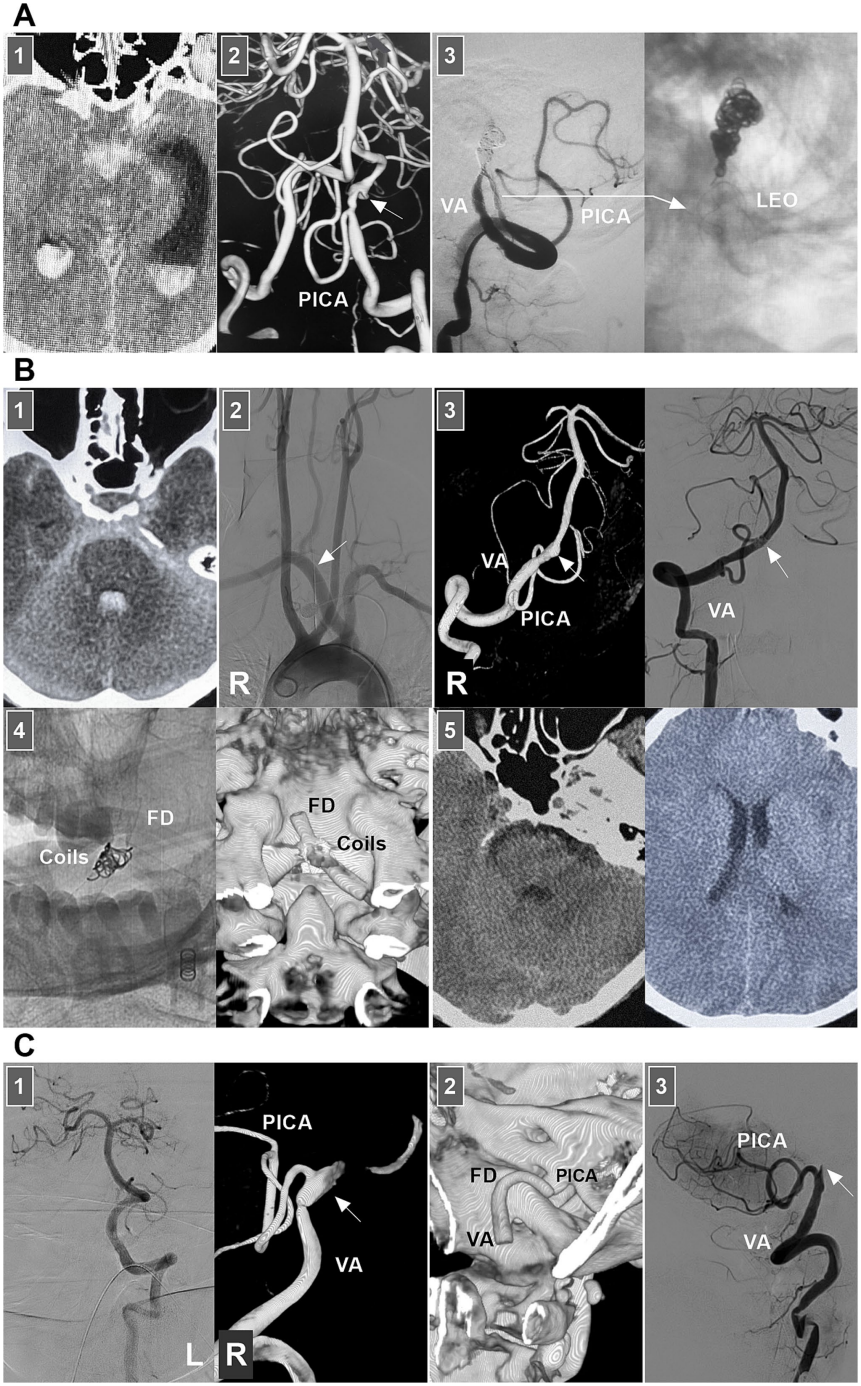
EVT of VA dissections. (A) Panel 1: CT showing SAH and intraventricular hemorrhage. Panel 2: DSA showing a ruptured VA dissection (arrow) above the PICA. Panel 3: DSA images (left and right panels) showing that the dissection was trapped and the PICA was stented by LEO deployment (long arrow). (B) Panel 1: CT showing SAH and intraventricular hemorrhage. Panel 2: DSA of aorta showing a right aberrant subclavian artery (arrow). Panel 3: DSA (left panel) showing a ruptured VA dissection (arrow) above the PICA, and DSA (right panel) showing the dissection (arrow) was embolized. Panel 4: X-ray image (left panel) and reconstructive Xper-CT (right panel) showing the FD and coils. Panel 5: Postoperative CT images (left and right panels) showing that intracranial hemorrhage absorbed. (C) Panel 1: DSA images showing that left VA was well-developed (left panel) and there was a right VA dissection (arrow) with PICA involvement (right panel). Panel 2: Reconstructive CT showing the FD deployment from PICA to VA. Panel 3: DSA showing the PICA obtained sufficient blood flow from the proximal VA, and the distal VA (arrow) occluded beyond the PICA after the FD deployment from PICA to VA. CT, computed tomography; DSA, digital subtraction angiography; EVT, endovascular treatment; FD, flow diverter; L, left; PICA, posterior inferior cerebellar artery; R, right; SAH, subarachnoid hemorrhage; VA, vertebral artery.
